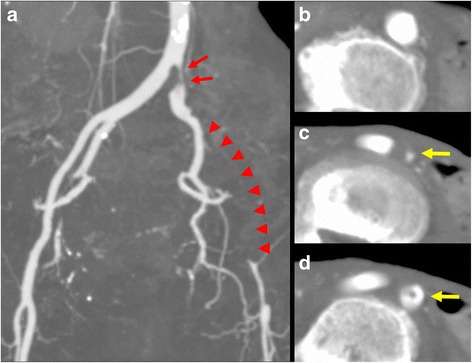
a Computed tomography angiography revealing severe stenosis of the left common iliac artery (*red arrows*) and total occlusion of the left external iliac artery (*arrowheads*). b-d Axial scan of the bifurcation showing a non-calcified plaque existing at the ostium of left CIA (*yellow arrows*)
